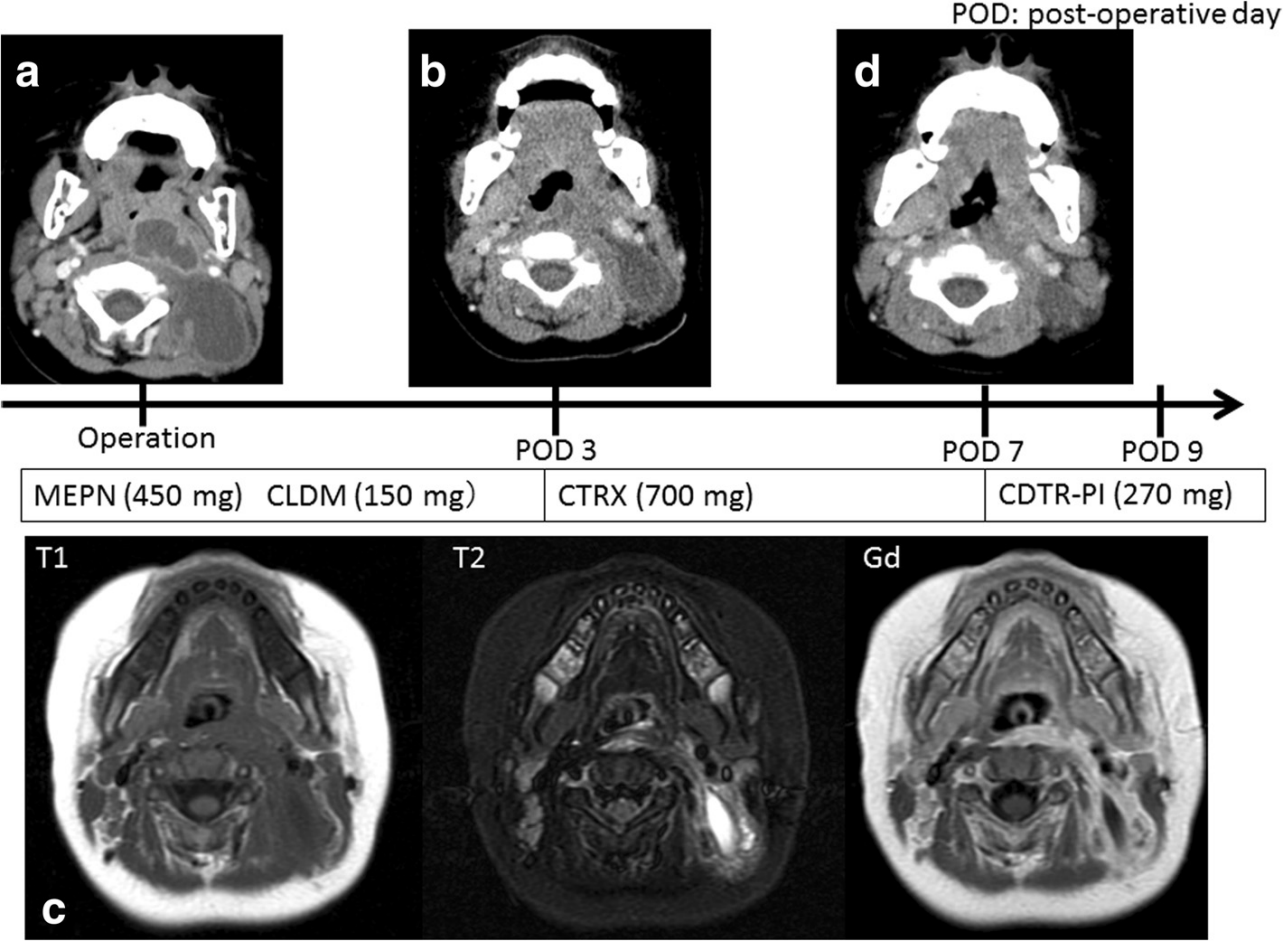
Postoperative progress. (a) Preoperative neck CT angiography image. (b) CT image on postoperative day (POD) 3, showing improvement of the retropharyngeal abscess but recurrence of the posterior neck swelling. (c) MRI on POD 3, showing a lesion with low signal intensity on T1-weighted images and high signal intensity on T2-weighted images, and an area of low signal intensity surrounded by an area of high signal intensity on post-gadolinium images. (d) CT image on POD 7, showing slight asymmetry between the right and left sides of the neck. MEPN, meropenem; CLDM, clindamycin; CTRX, ceftriaxone; CDTR-PI, cefditoren-pivoxil.
